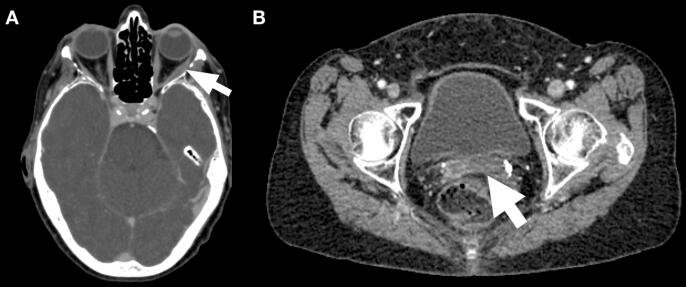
(A,B) Axial post-contrast CT images demonstrate treatment response. (A) Residual swelling of the left lateral rectus muscle (arrow) with resolution of previously visualized discrete mass. (B) Likewise, marked decrease in size of the primary cervical tumor (arrow).
